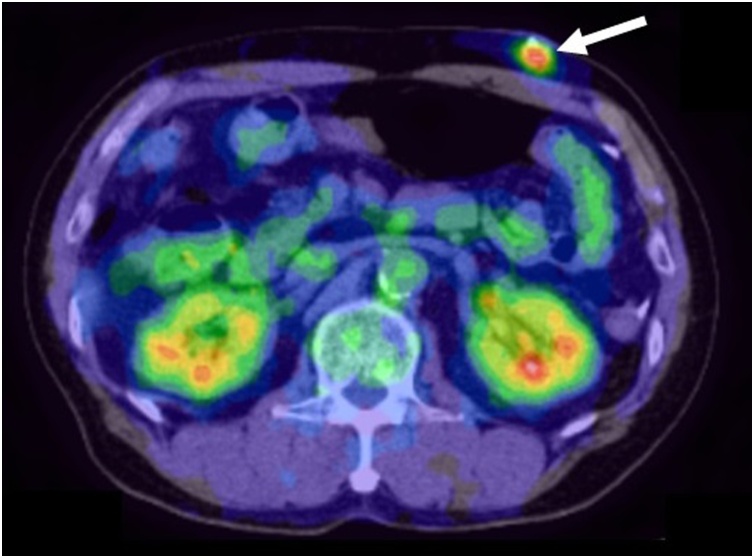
FDG-PET/CT scan showed FDG accumulation on the mass.
